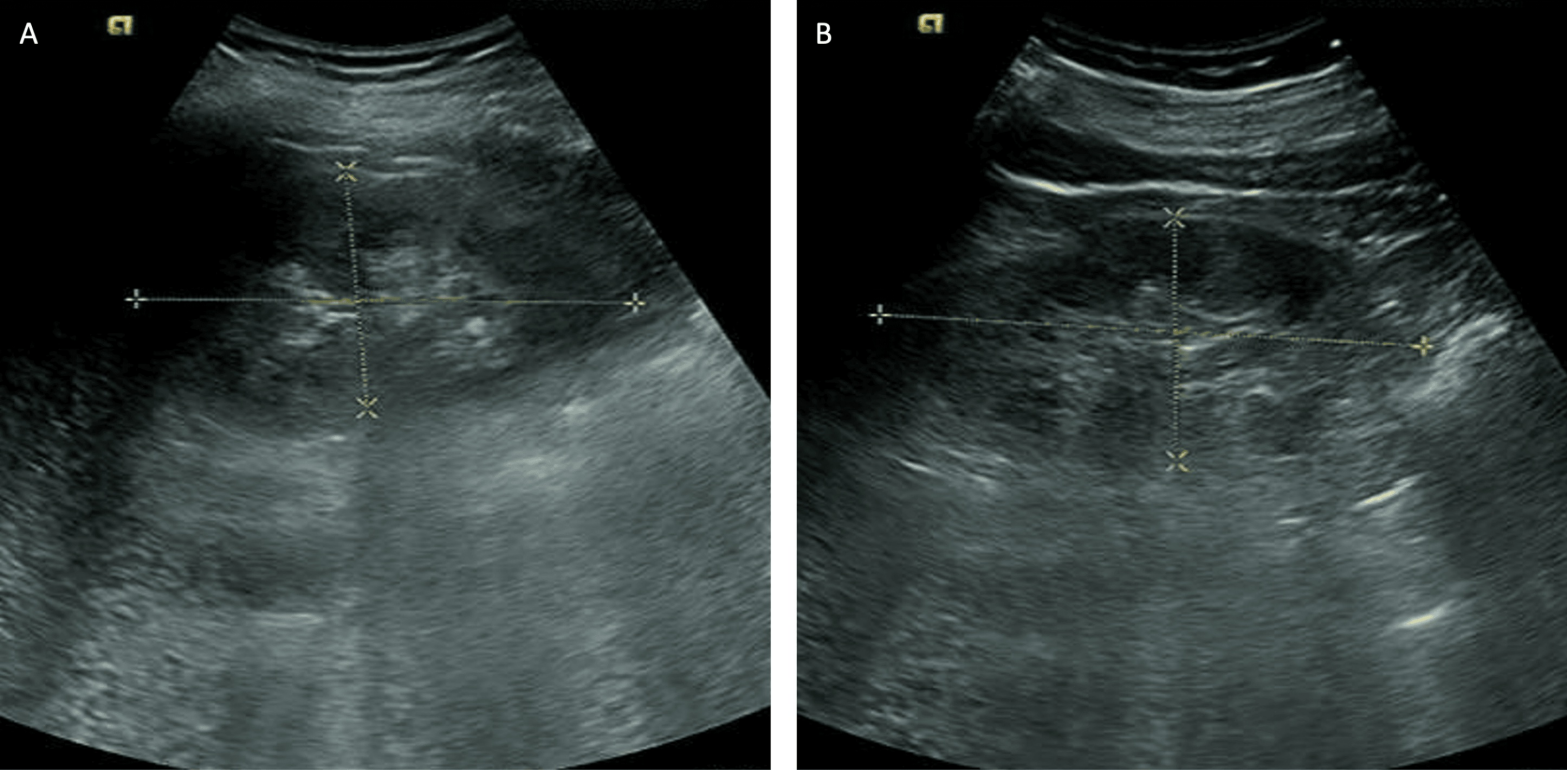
Renal ultrasound revealed diffuse lesions in both kidneys. (A) The right kidney. (B) The left kidney
